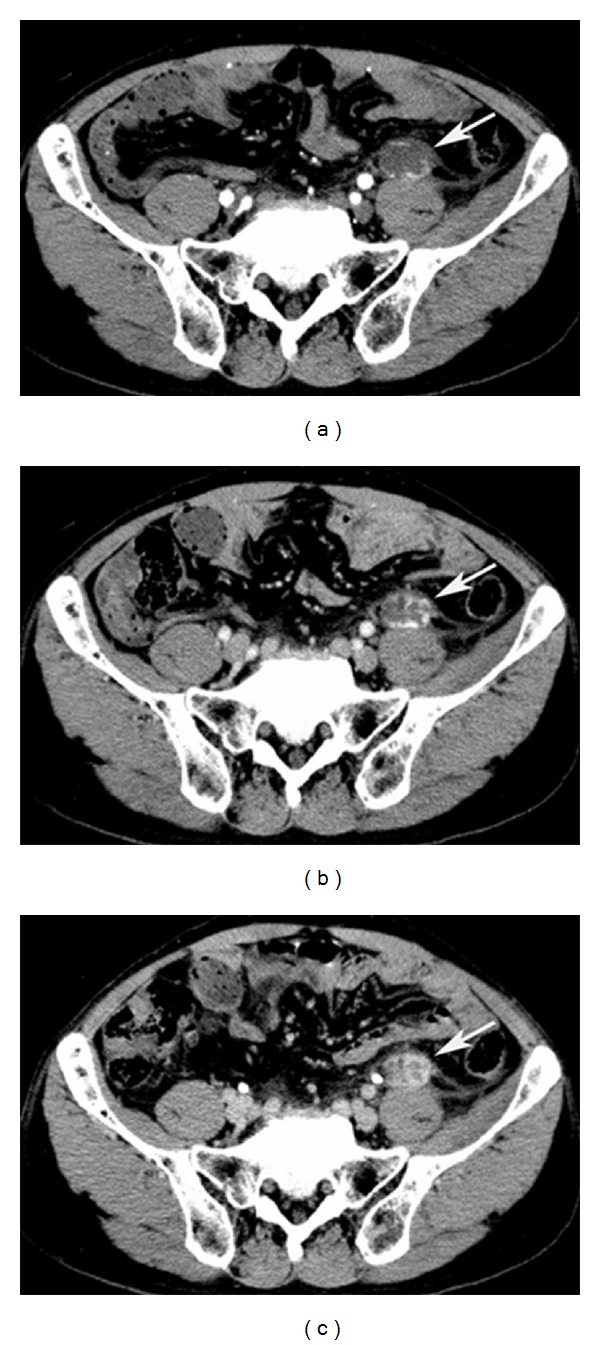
Dynamic contrast enhanced computed tomography. During the arterial phase (a), lesion demonstrates an initial peripheral nodular, with subsequent centripetal progressive filling-in pattern during venous (b) and equilibrium phase (c) (arrow).
